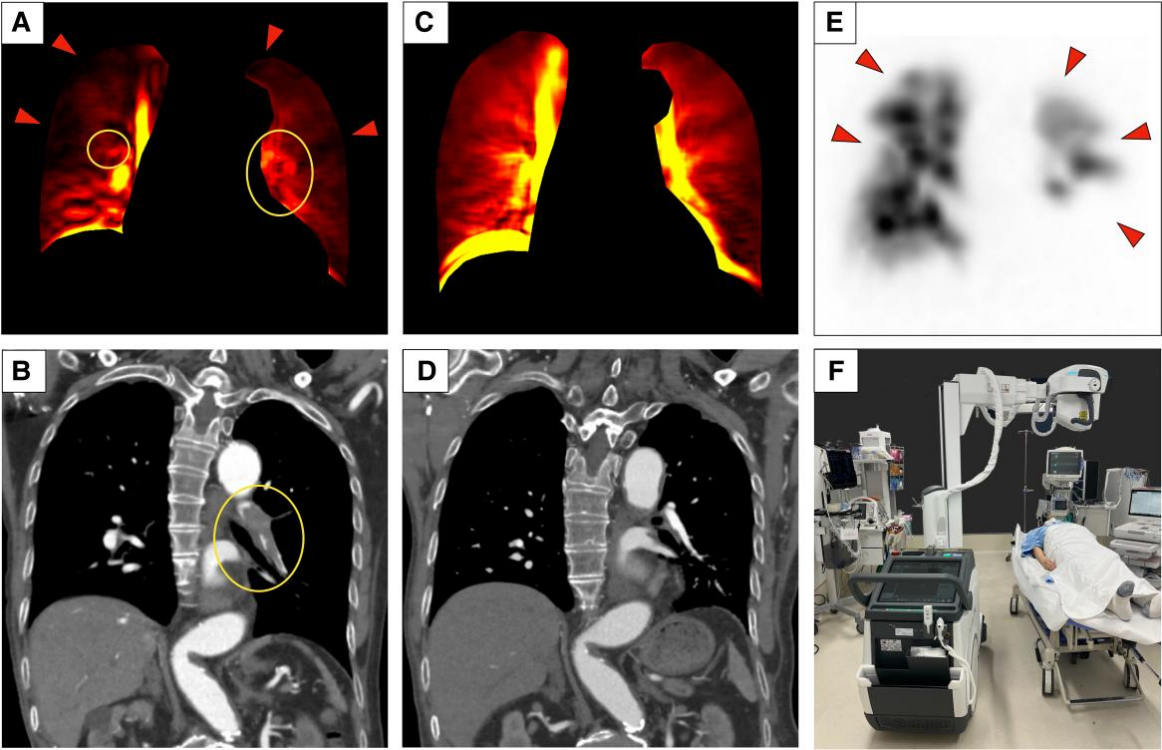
A 79-year-old man with nausea visited the emergency department (ED) by ambulance with decreased oxygen saturation. No abnormalities were noted on computed tomography (CT), performed without contrast due to his renal impairment. Given the elevated D-dimer level, portable dynamic chest radiography (DCR) was performed in the ED using a mobile radiography system (Aero DR TXm01, KONICAMINOLTA, Japan) (*Panel F*) to assess suspected acute pulmonary embolism (PE). One of the modes from DCR imaging analysis (*Panel A*) identified diminished blood flow signals in the proximal pulmonary arteries (yellow circle) and in lung fields (red arrowheads), suggesting PE. On the same day, perfusion scintigraphy confirmed perfusion defects in similar areas of lung fields (*Panel E*, red arrowheads). After renal function improvement, CT pulmonary angiography revealed multiple PEs (*Panel B*, yellow circle) the next day. Post-treatment DCR revealed improved blood flow signals without defects (*Panel C*). Different appearance of pre-treatment image relative to normal one is reminiscent of the ‘melting wings of Icarus’. Follow-up CT pulmonary angiography confirmed disappearance of PEs (*Panel D*).
